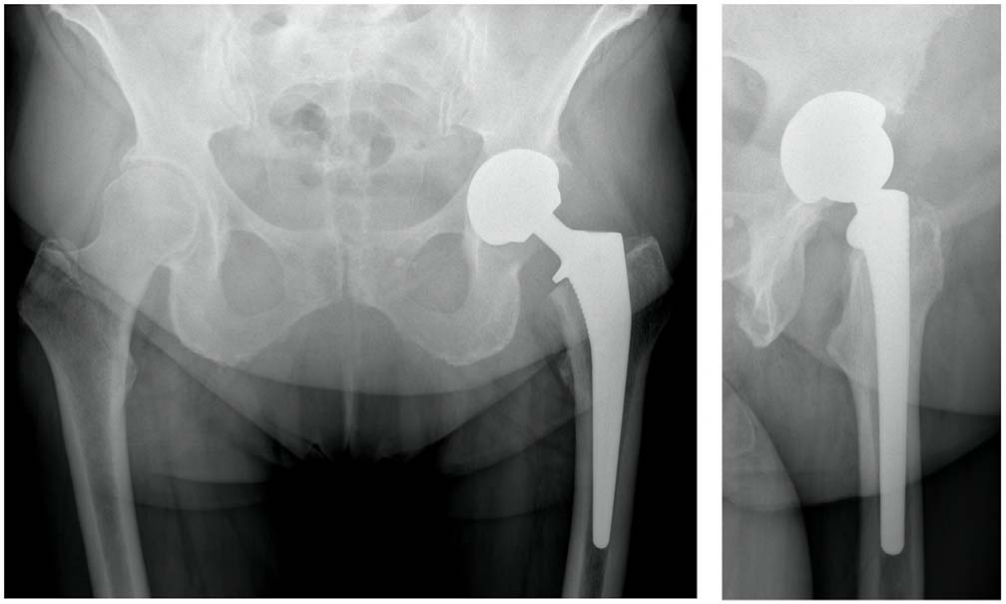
Antero-posterior pelvic radiograph and profile left hip radiograph of a patient with psoas pain, despite a satisfying cup positioning without cup overhang.
